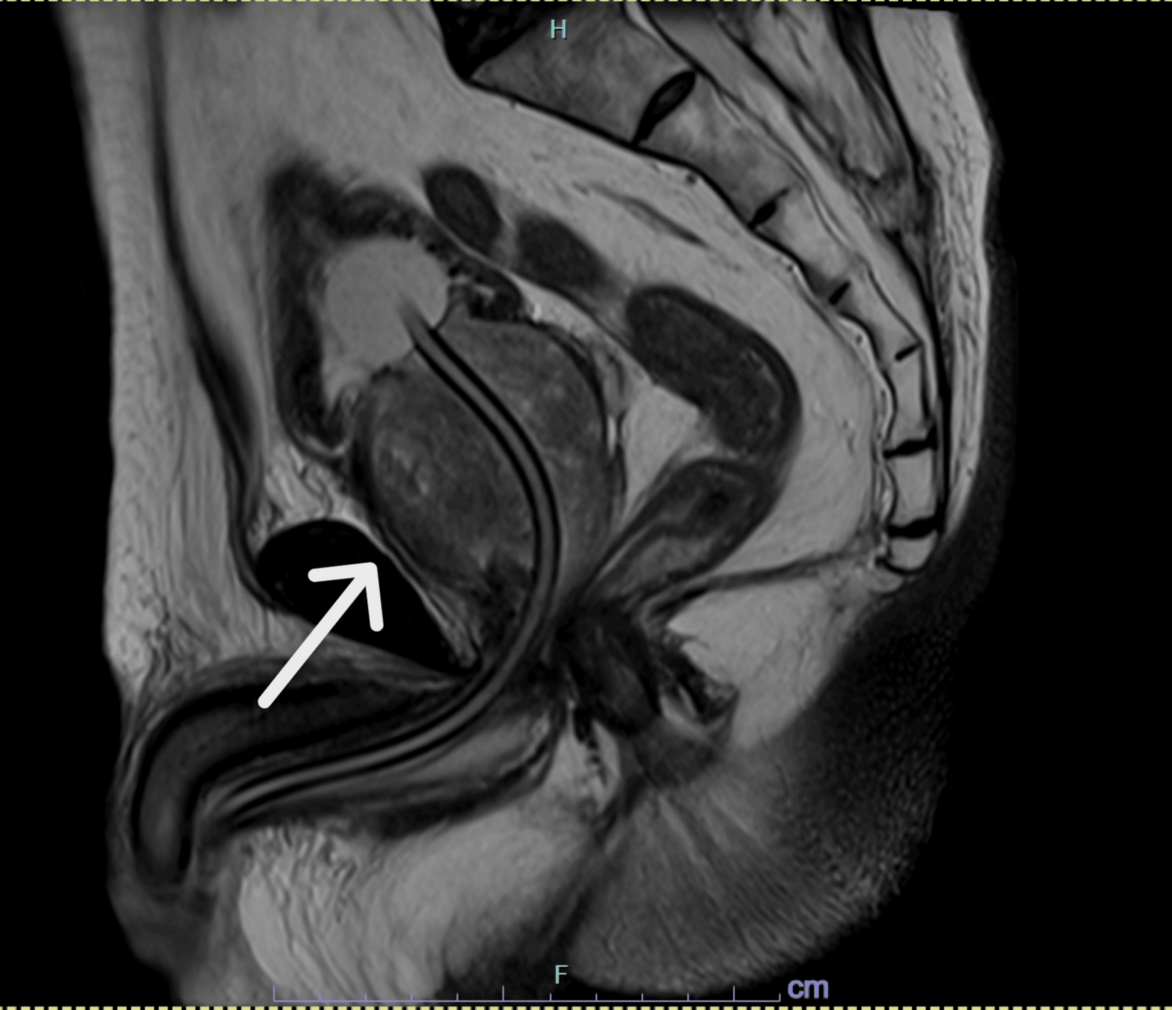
MRI T2 sagittal view of the massive prostate when a Foley catheter was placed
